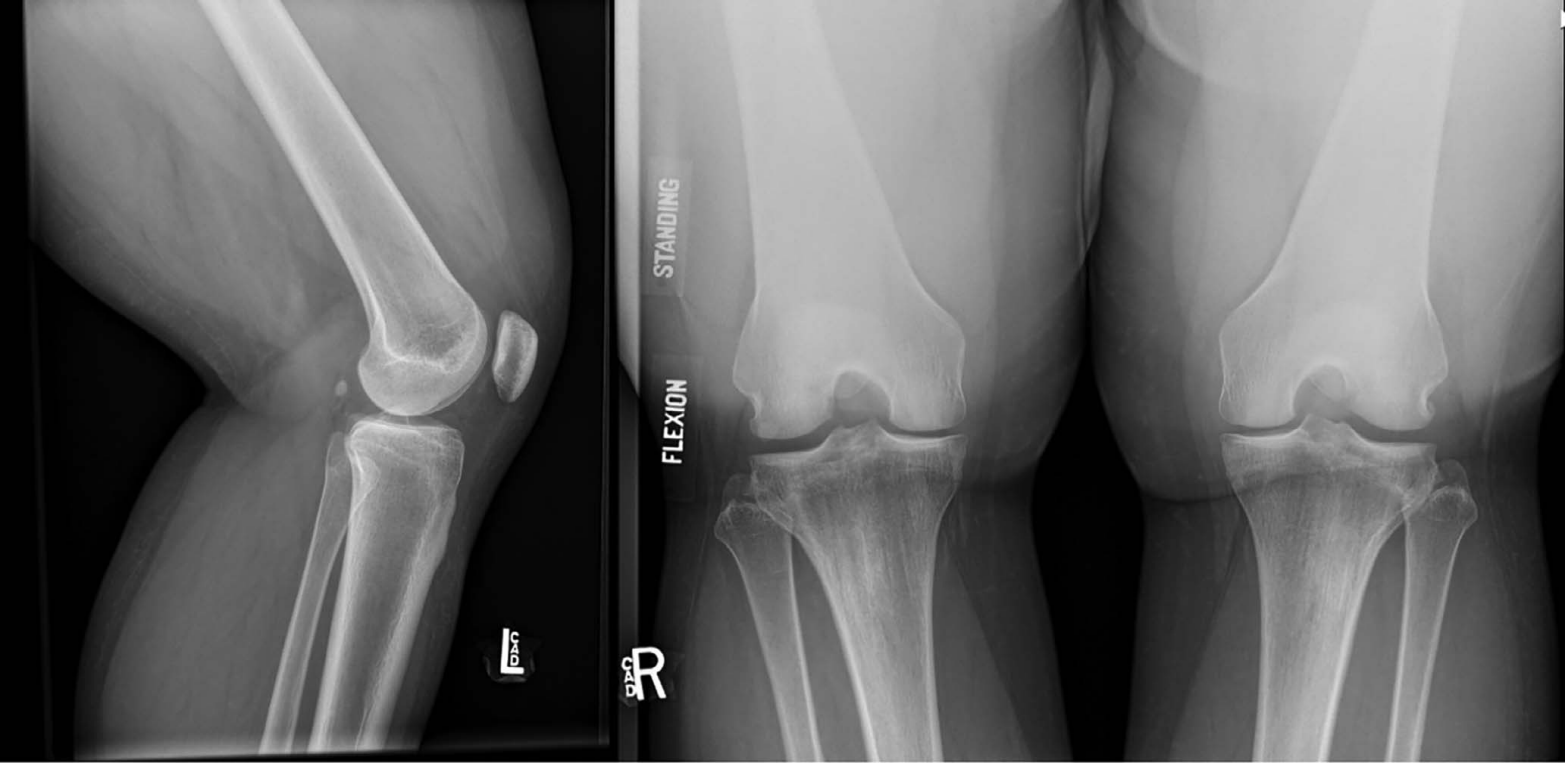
Lateral and standing flexion plain radiographic views of the left knee show no evidence of fracture, dislocation, or degenerative changes.
